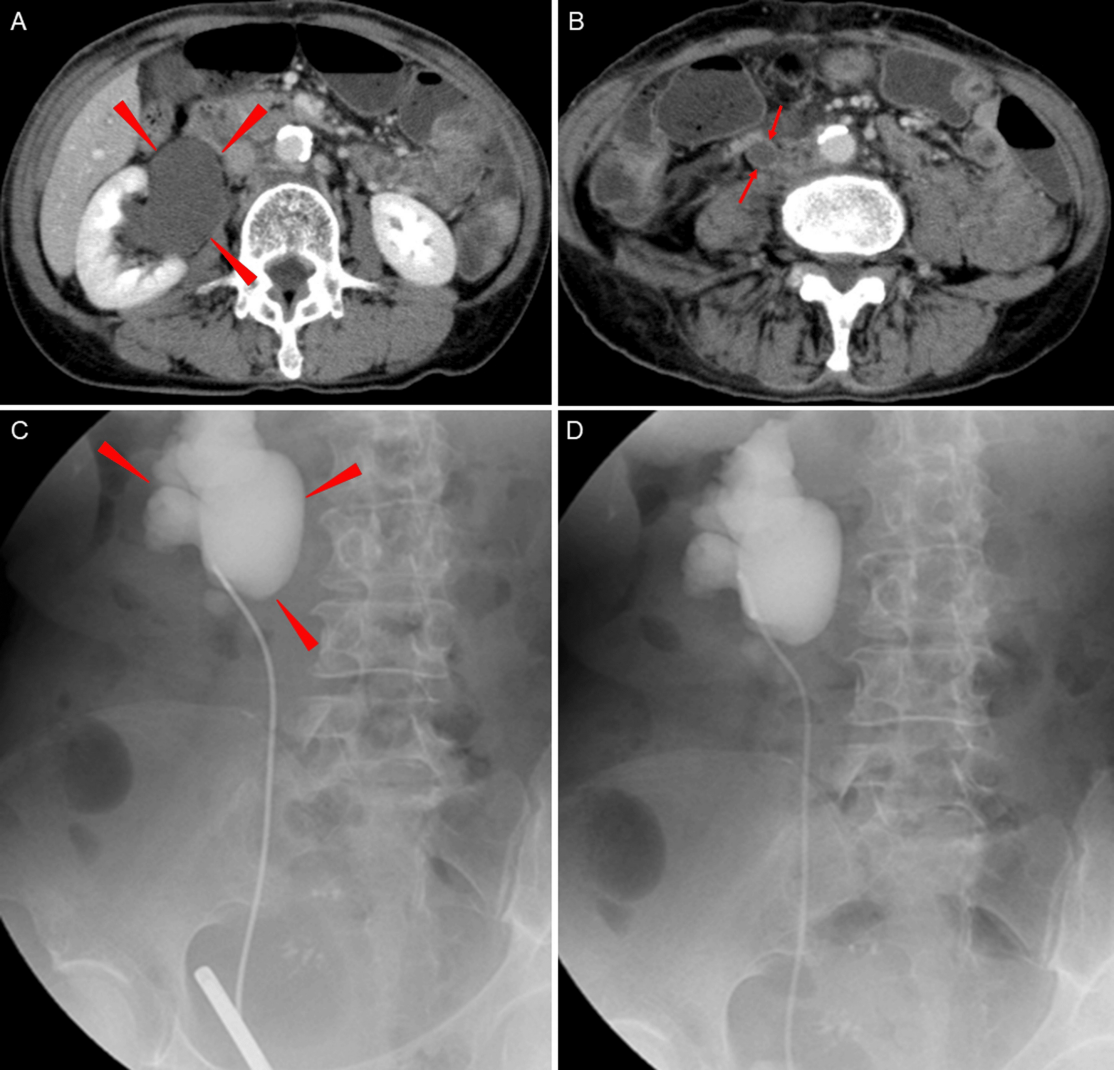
Radiological findings of advanced hydronephrosis and ureteral involvement caused by retroperitoneal dissemination, and its management with double-J stent placement (A) Contrast-enhanced abdominal computed tomography showing marked right hydronephrosis and hydroureter (arrowheads), accompanied by retroperitoneal dissemination surrounding the kidney. (B) The ureter coursing through the area of retroperitoneal dissemination demonstrates long-segment ureteral wall thickening and luminal narrowing (arrows). (C) Retrograde pyelography revealing no fixed obstruction of the right ureter but demonstrating marked hydronephrosis (arrows). Based on these findings, malignant ureteral obstruction due to retroperitoneal dissemination was diagnosed. (D) A 24 cm, 6 Fr double-J stent was placed smoothly and without resistance, resulting in appropriate drainage
